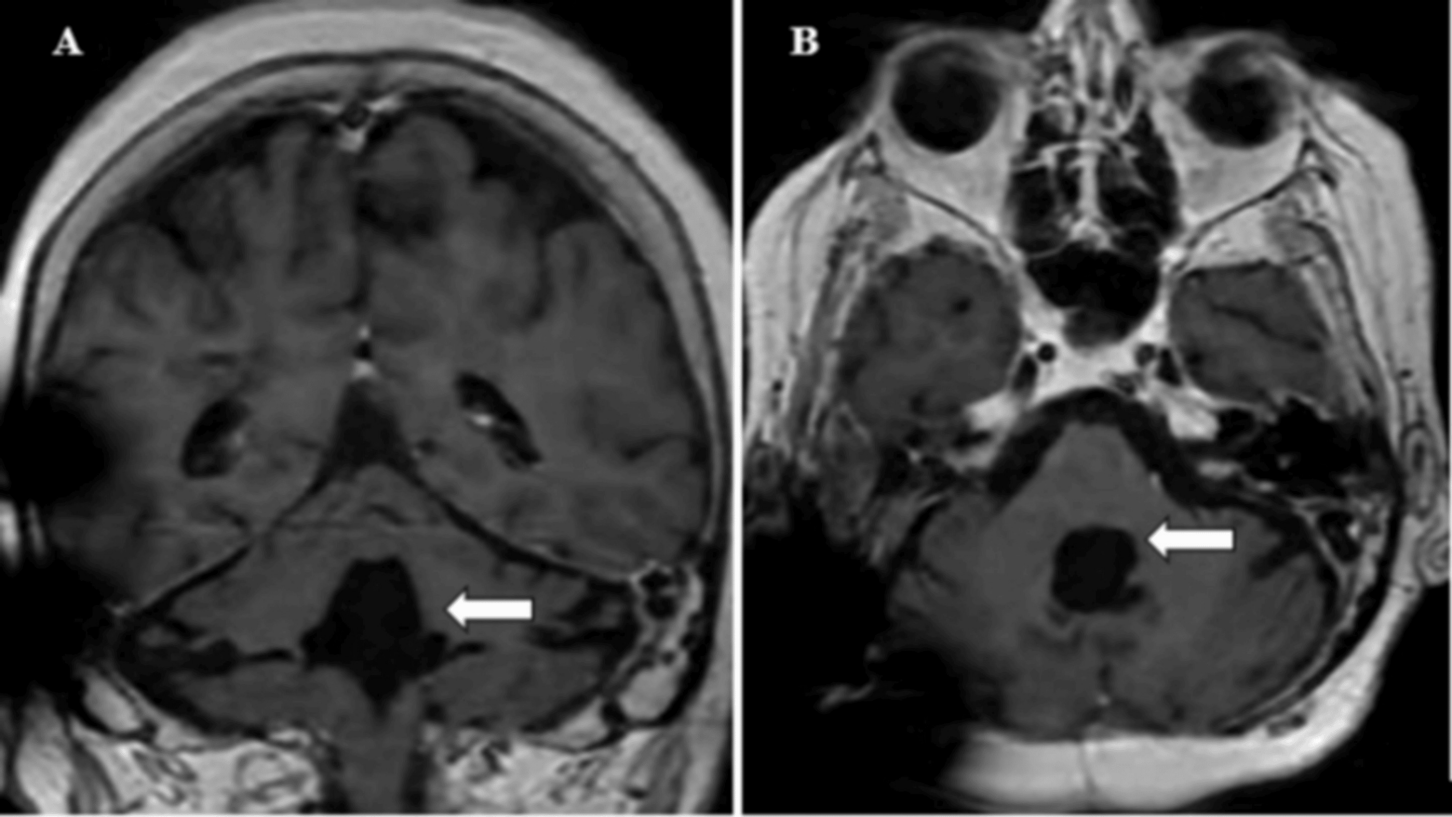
Three-year postoperative follow-up contrast-enhanced T1-weighted MRI in coronal (A) and axial (B) views (arrow). The images demonstrate postoperative changes with no evidence of lesion recurrence in the posterior fossa. A compensatory dilation of the fourth ventricle is present. The lateral and third ventricles are normal in size, with no hydrocephalus.
